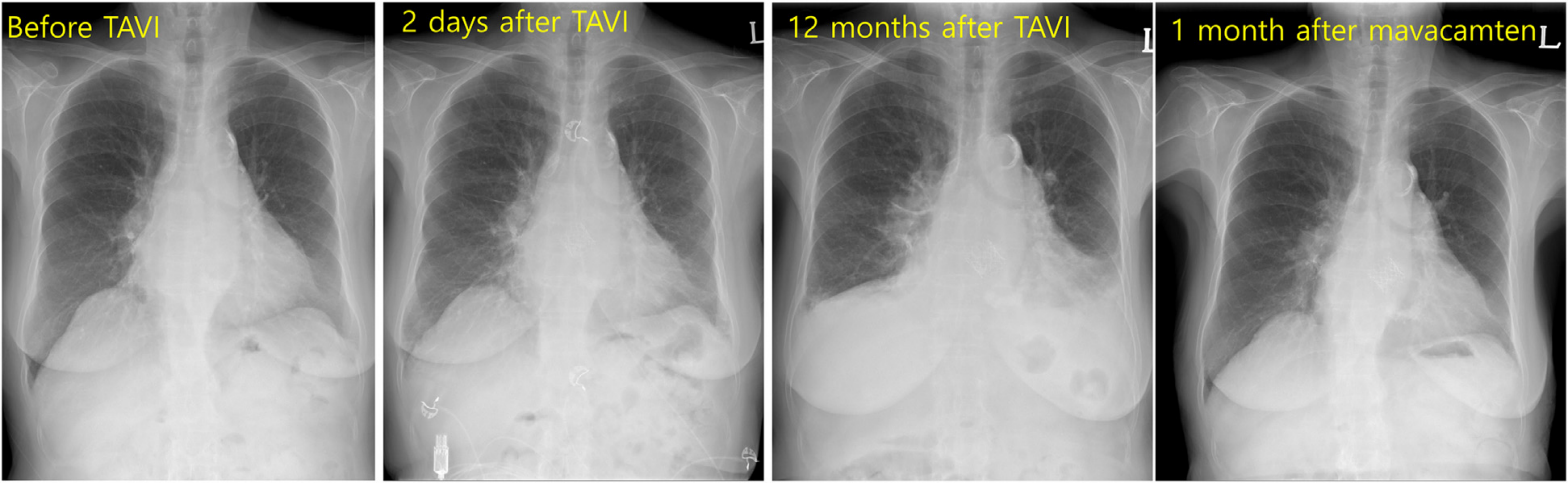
Chest X-Ray Serial chest x-ray showed late development of pulmonary congestion and bilateral pleural effusion despite medical treatment. The addition of mavacamten led to a significant normalization of heart size and the disappearance of bilateral pleural effusion. Abbreviation as in Figure 1.
